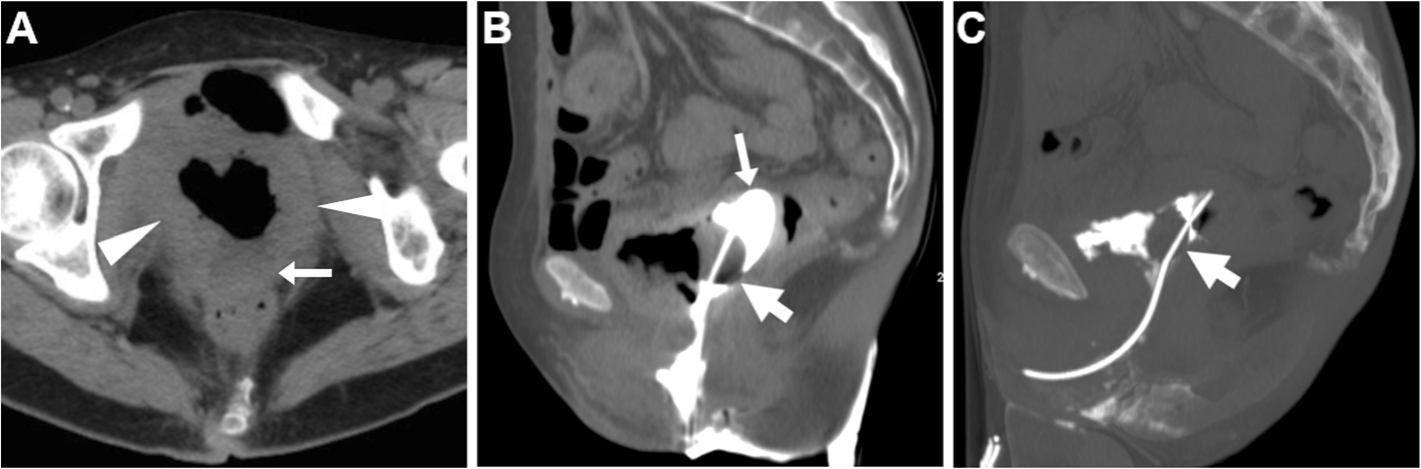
CT-vaginography in a patient with advanced bladder and urethral undifferentiated carcinoma invading the vagina, seen as marked circumferential mural thickening (arrowheads in precontrast CT a). After introduction and inflation of the Foley catheter (thick arrows) in the vagina, injection of CM medium allows opacification and distension of the vagina (arrow at vaginal dome in b) and subsequent CM flow anteriorly into the urinary bladder (in c)
